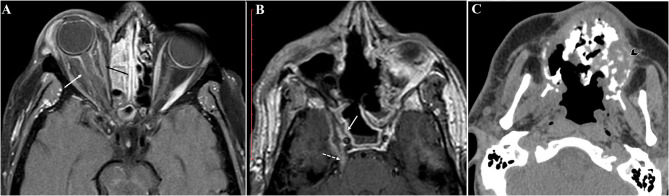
Radiological findings in our rhino-orbital-cerebral mucormycosis (ROCM) cases. Axial postcontrast T1WI showing (A) orbital infiltration with optic nerve sheath inflammatory changes (white arrow), the extra ocular muscles are hypoenhancing (black arrow) secondary to ischemic changes, and (B) cavernous sinus infiltration with lack of expected enhancement (solid white arrow). Perineural spread of the fungus is seen along the trigeminal nerve (dashed white arrow). (C) Axial non-contrast CT reveals destructive sinonasal soft tissue mass with fragmented bone and air loculi secondary to osteonecrosis (arrowhead).
